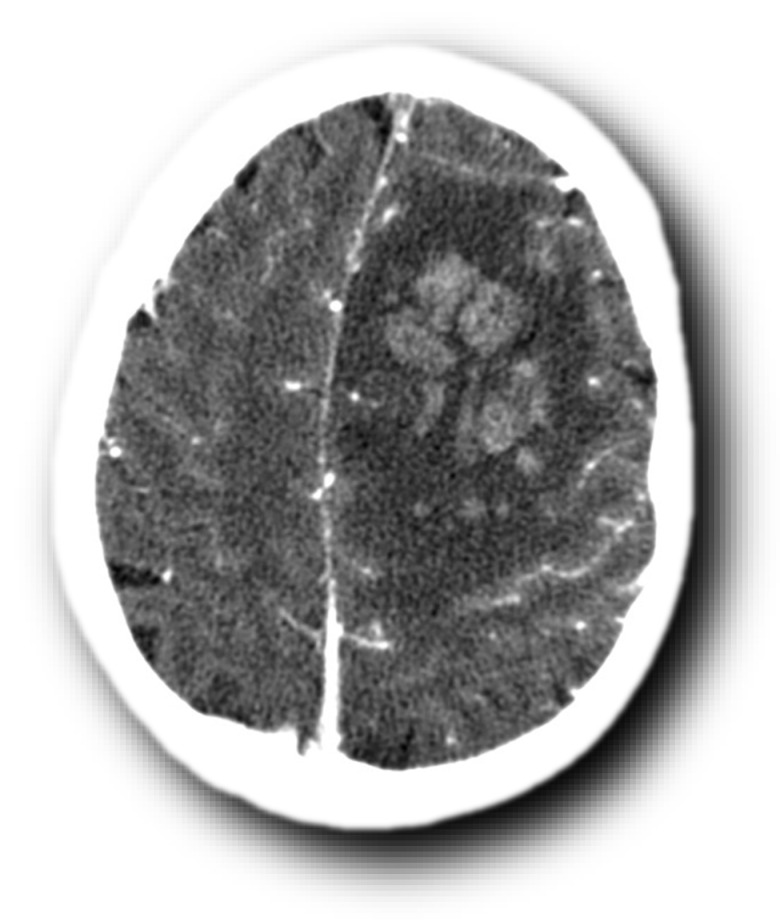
Left frontopolar venous hemorrhagic softening on CT.
